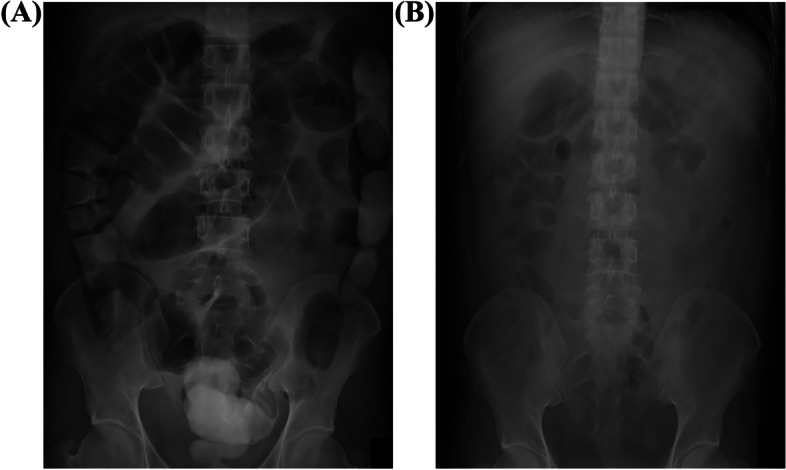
Changes in abdominal radiographs after treatment. Abdominal radiographs on admission (A) and hospital day 6 (B)
